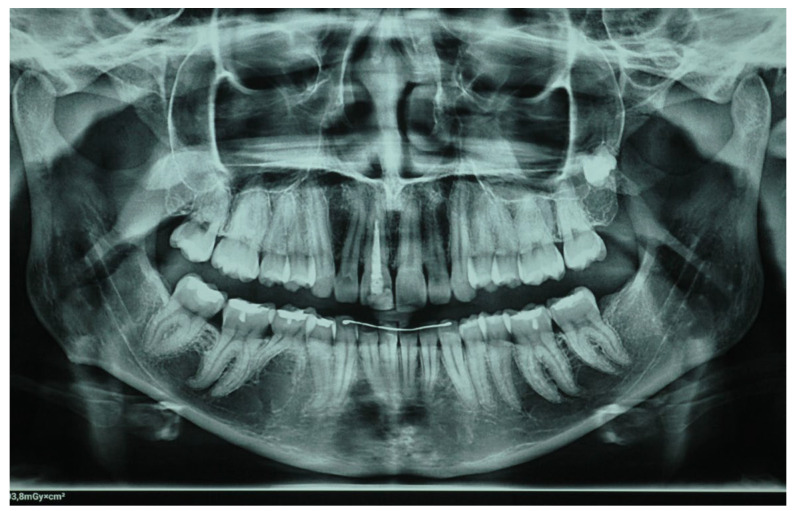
The panoramic X-ray of the patient.
